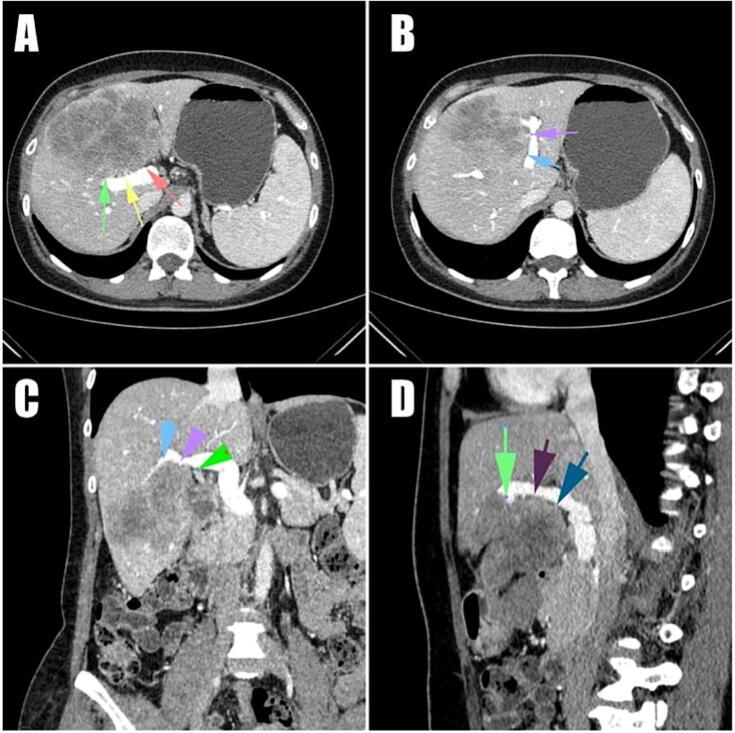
CT scans before the CTx.
